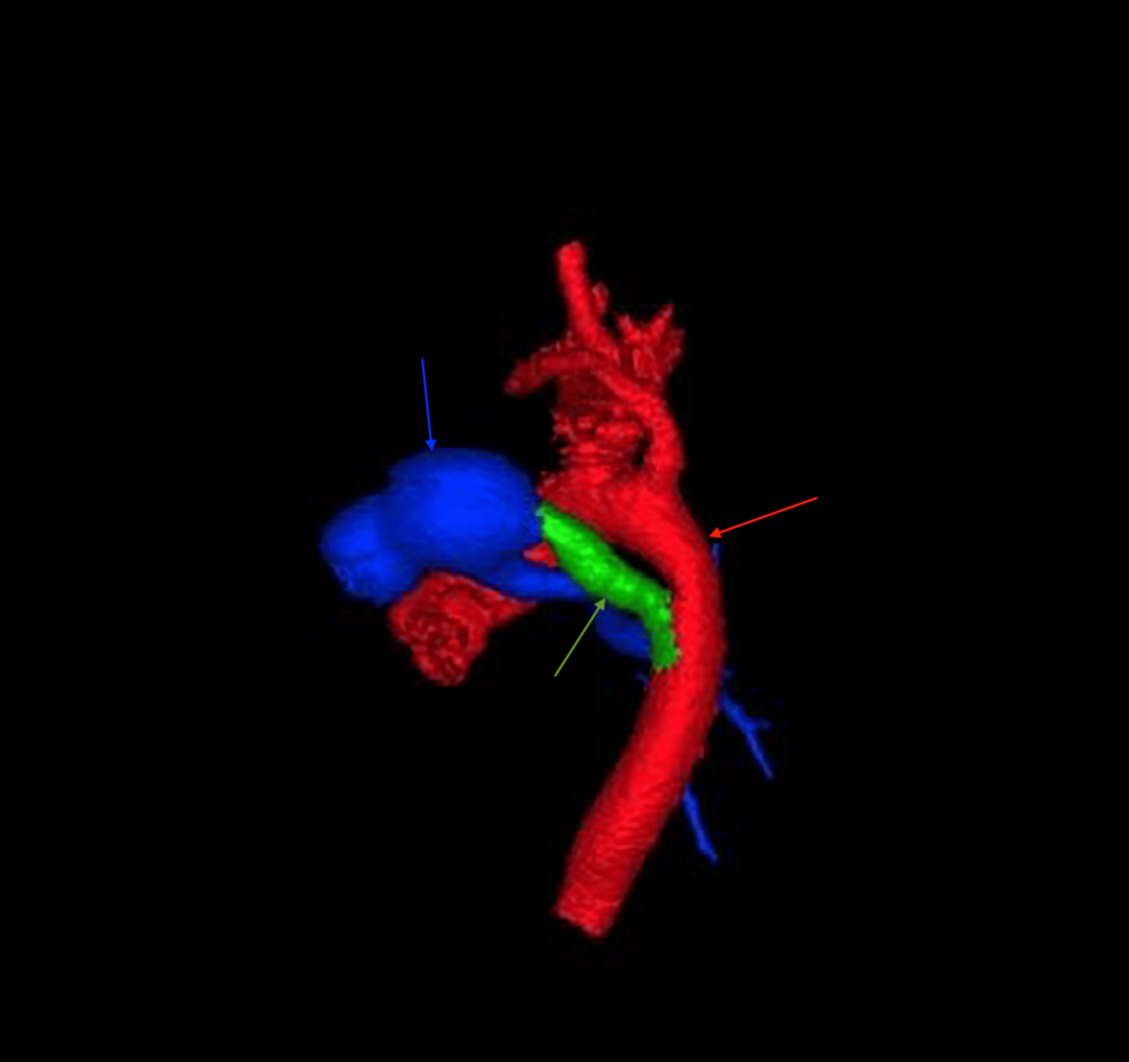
Three-dimensional computed tomography angiography reconstruction (left lateral view) demonstrating patent ductus arteriosus (green arrow) The patent ductus arteriosus connects the hypoplastic right pulmonary artery (blue arrow) to the descending thoracic aorta (red arrow).
